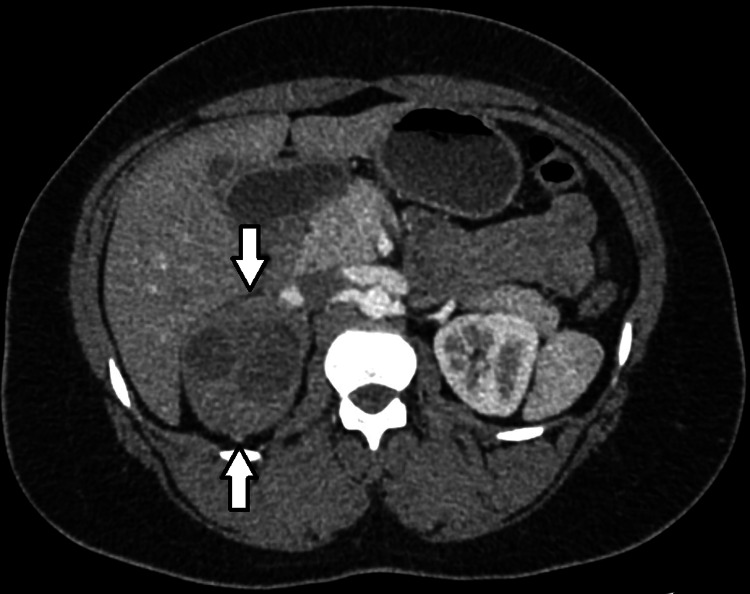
CT abdomen with contrast (case 1) - right-sided mixed semi-cystic mass (white arrows) lying inferior to liver and superior to but not arising from the right kidney. CT: computed tomography
